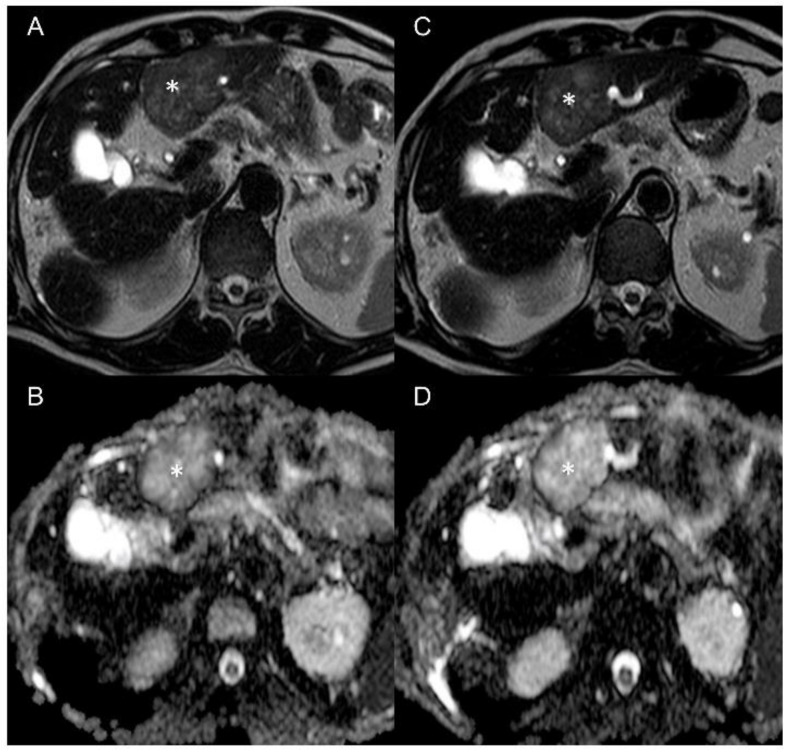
Imaging of patient 16 with colorectal carcinoma and liver metastases (marked with an asterisk) before (A,B) and after (C,D) the second cycle shows an increase in ADC value (B + D) and changes in T2 weighted imaging (A + C). These morphological changes can be further assessed with the multiparametric MRI protocol.
